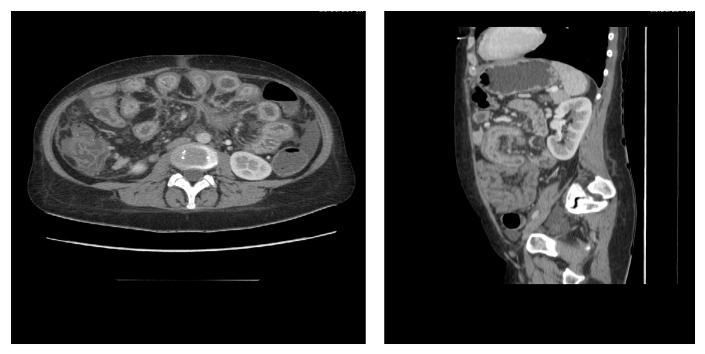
Abdominal computed tomography (CT). CT of the abdomen shows ascites. Liver, pancreas, spleen, and kidneys look normal. Thickened wall of sigmoid and ascending colon, cecum, and a significant part of the ileum, inflammatory changes. Numerous, enlarged mesenteric lymph nodes.
